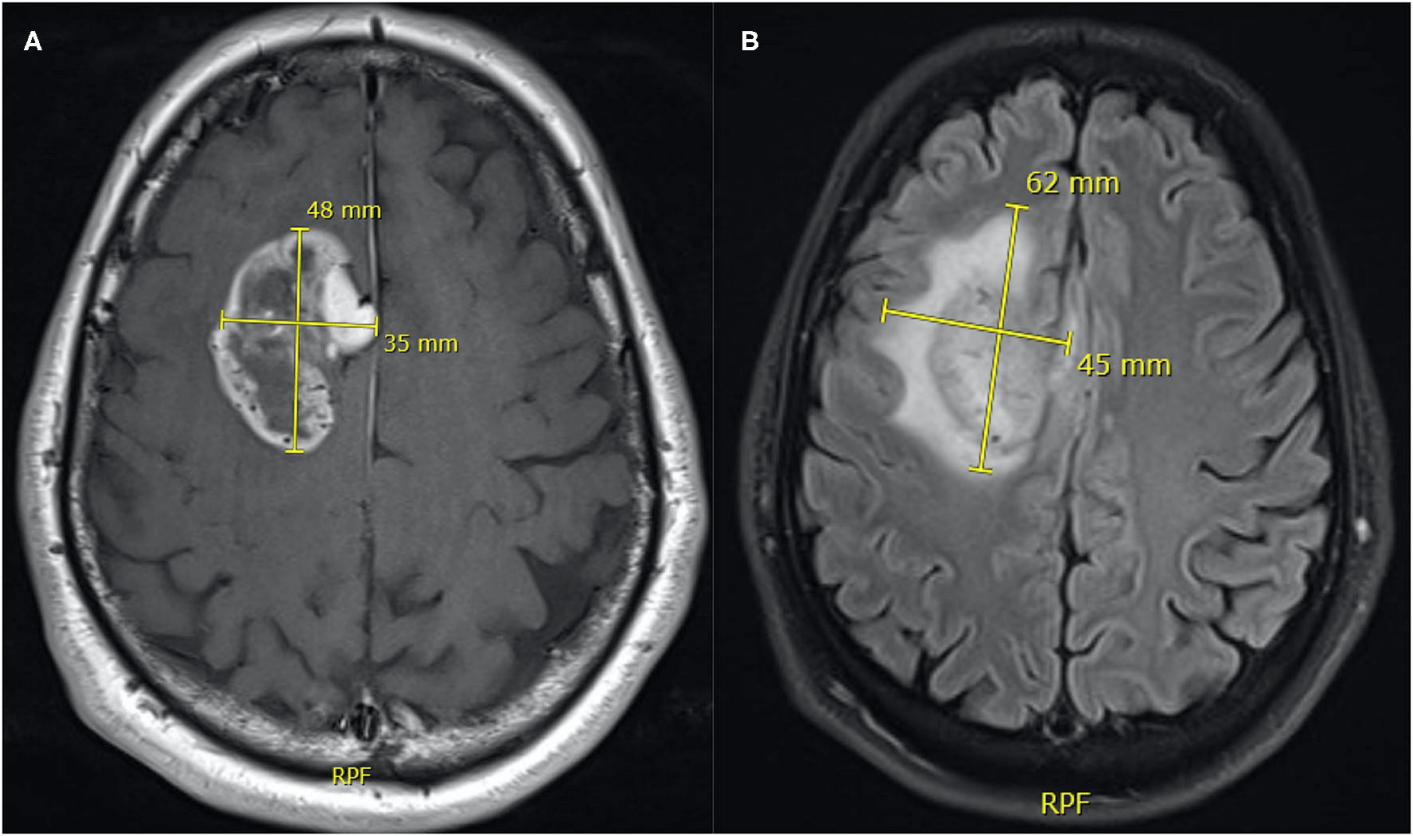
Representative measurements of tumor size (A) and FLAIR-T_2_
